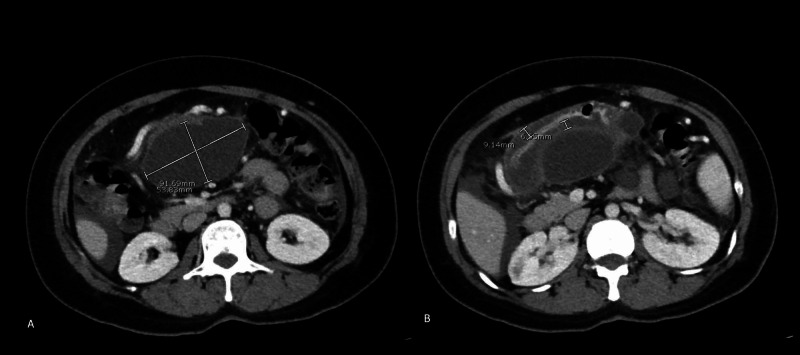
(A): Initial CTAP showing a large dominant fluid collection anterior to the pancreas, extending along the posterior aspect of the mid and distal stomach, arising from the neck of the pancreas, measuring 9.2 x 5.4 cm, with an estimated volume of 250 ml (marked with white pointers); (B): Peripancreatic edema with inflammatory stranding in the pancreatic tail (marked with white pointers) CTAP: computed tomography of the abdomen and pelvis; cm: centimeter; ml: milliliter
